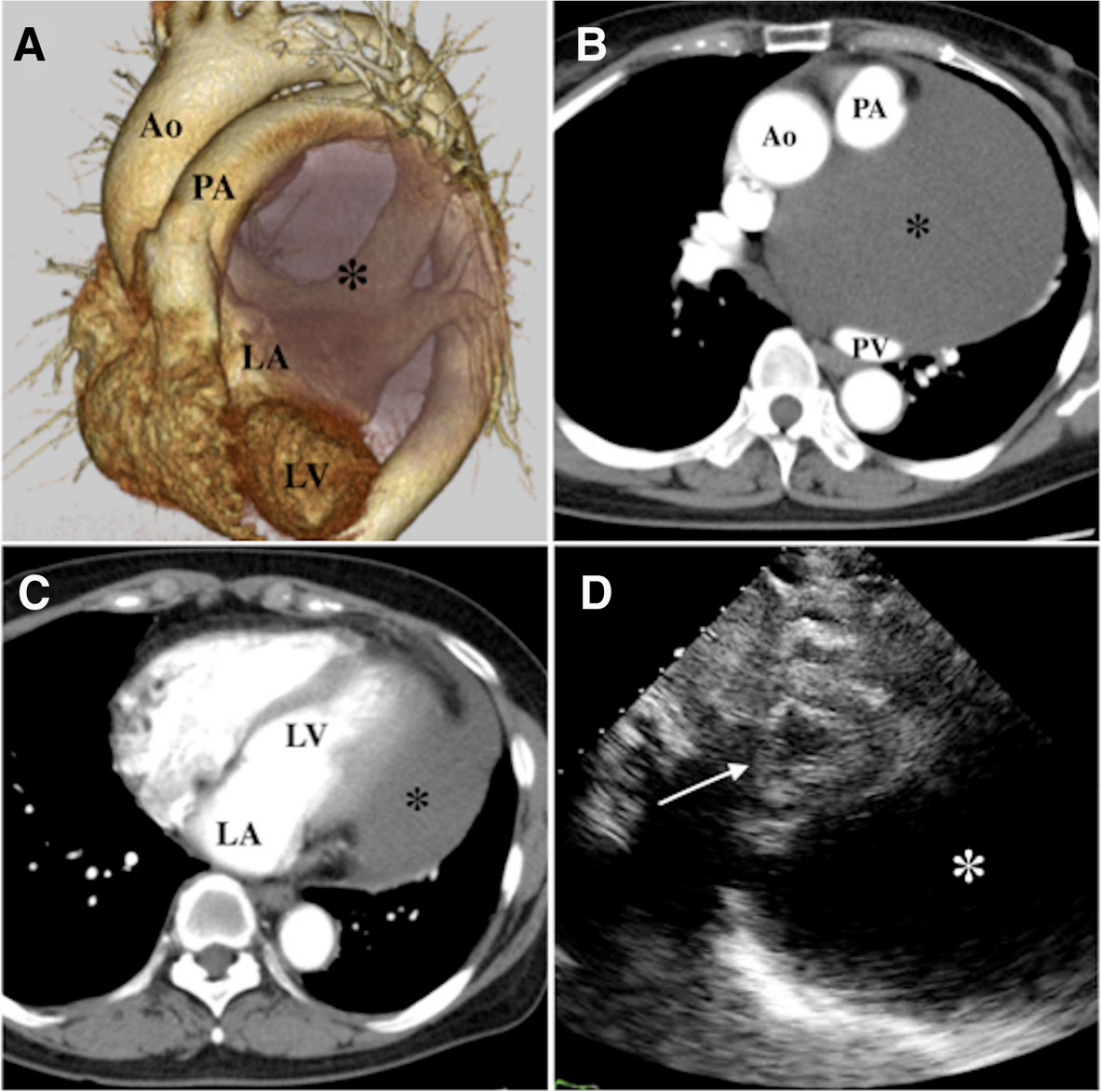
a Volume-rendered computed tomography showing a giant cyst (12 × 10 cm in diameter; asterisk) compressing the left atrium and ventricle. Ao aorta, PA pulmonary artery, PV pulmonary vein, LA left atrium, LV left ventricle. b Preoperative enhanced computed tomography in the horizontal view showing adhesion of the cyst to the pulmonary artery and pulmonary vein. c Computed tomography in the horizontal view showing the cyst compressing the left ventricle. d Echocardiography showing the cyst (asterisk) compressing the left ventricle (white arrow)
